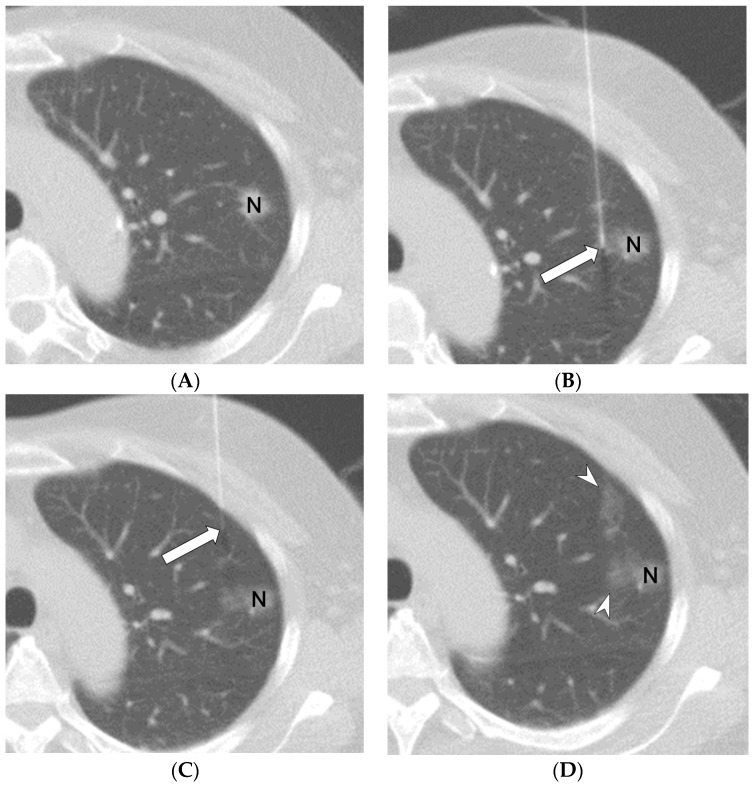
A 65-year-old woman undergoing CT-guided localization with PBV dye. Preprocedural chest CT revealed a 1.0-cm part-solid nodule (N) in the left upper lobe ((A), CT axial view). Two PBV dye doses were then slowly injected, with the first localization site being placed near the deepest point of the target lesion (N) in the lung parenchyma ((B), white arrow) and the second site near the pleural surface of needle entry ((C), white arrow). PBV dye was then administered, resulting in the nodule (N) being clearly marked ((D), white arrowheads).
